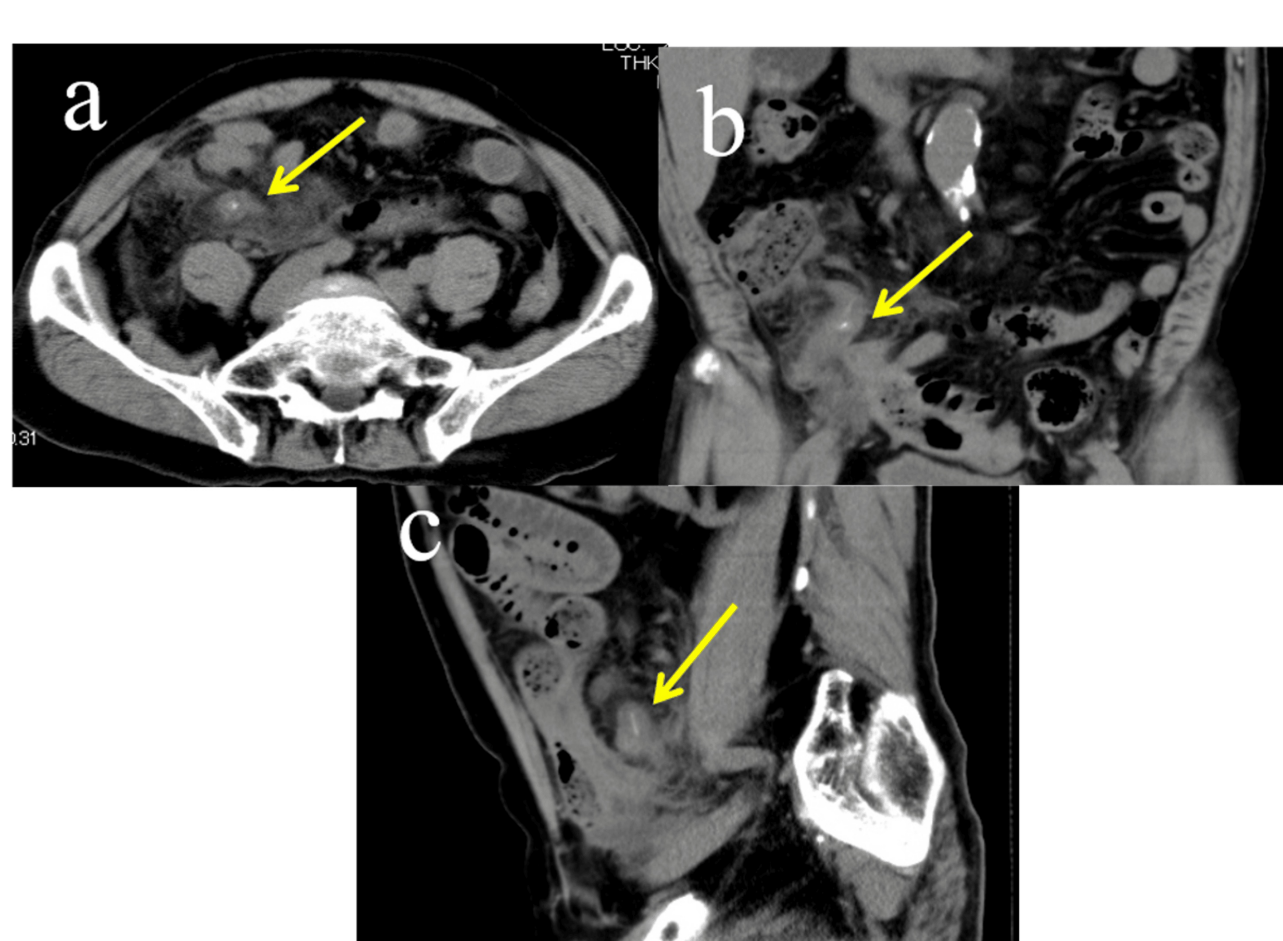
Computed tomography (CT) images of the abdomen. (a) Axial view. (b) Coronal view. (c) Sagittal view. The appendix was swollen to 16 mm in diameter, with a hyperdense shadow (yellow arrow) observed within, confirming acute appendicitis with appendicolith. Significant inflammatory spread to the surrounding tissues was noted, but no abscess formation was identified.
